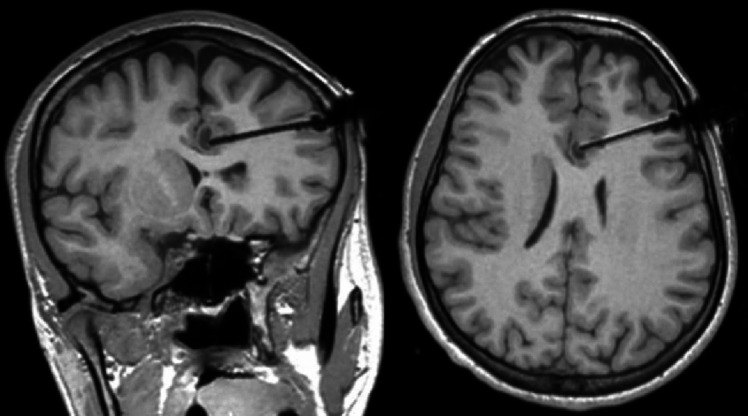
Intraoperative MRI of case #3 demonstrates the laser catheter pushing on the capsule of the lesion without penetrating it
